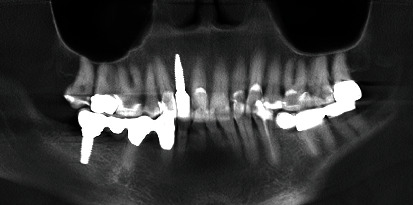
CB-CT after completion of the surgical and implant prosthetic rehabilitation.
